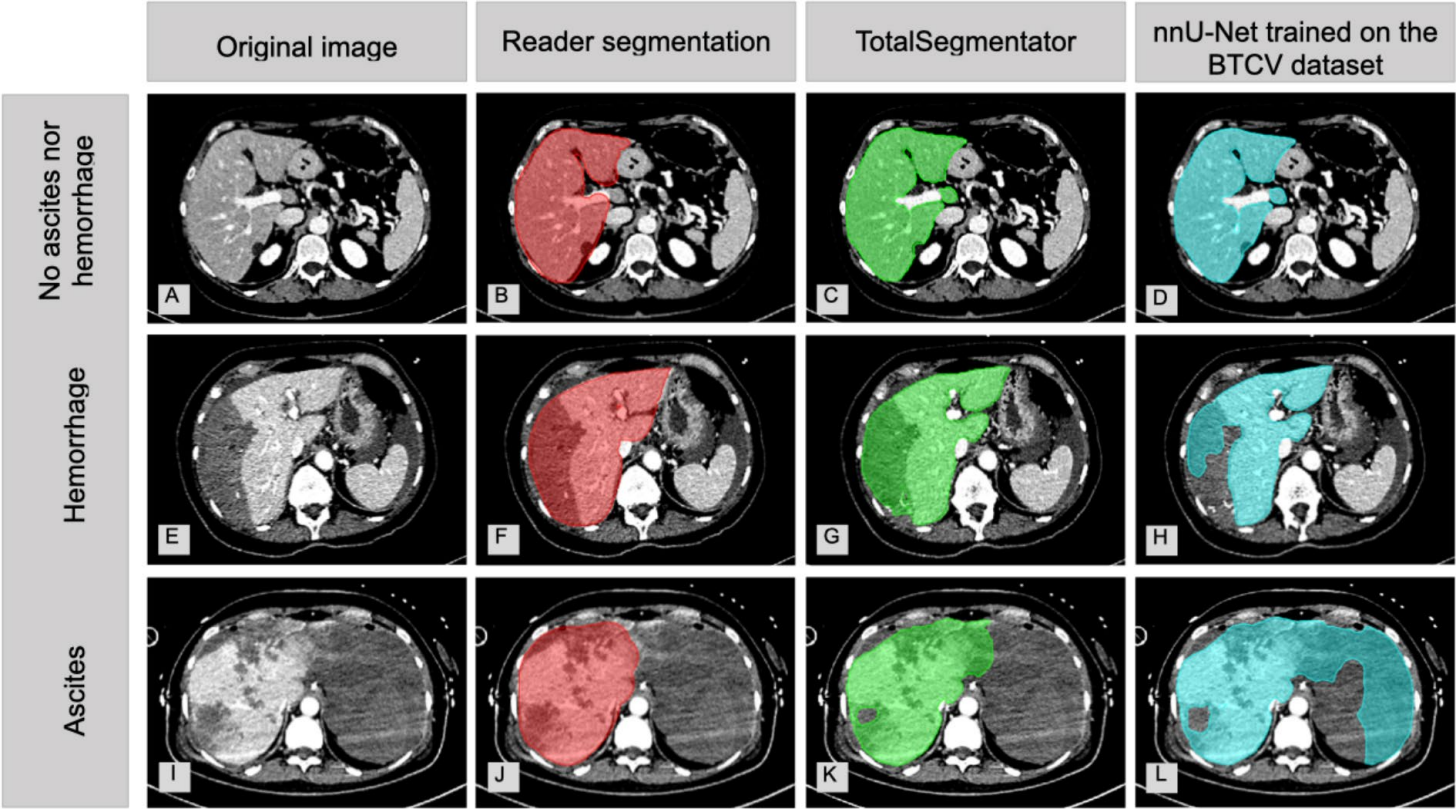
Side-by-side comparison of baseline CT images (A, E, I), manual human reader segmentations (B, F, J), automated TotalSegmentator segmentations (C, G, K), and automated segmentations using a nnU-Net trained on the BTCV dataset (D, H, L) in a non-fluid case (A–D), a case with liver hemorrhage (E–H), and a case with ascites (I–L)
